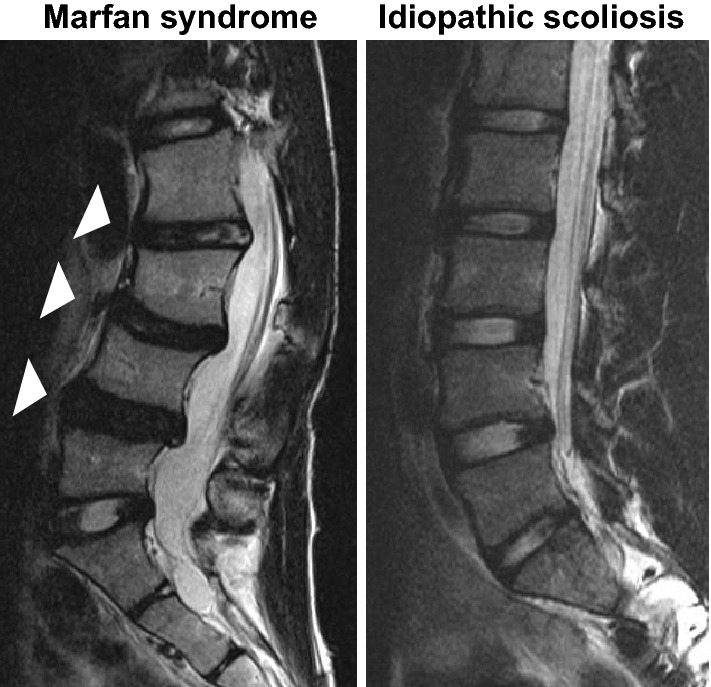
Representative preoperative magnetic resonance imaging (MRI) of the lumbar spine in patients with Marfan syndrome and idiopathic scoliosis. Representative preoperative MRI of the lumbar spine in patients with Marfan syndrome (left) and idiopathic scoliosis (right). Both cases were 14-year-old boys. White arrowheads indicate the degenerated intervertebral discs.
